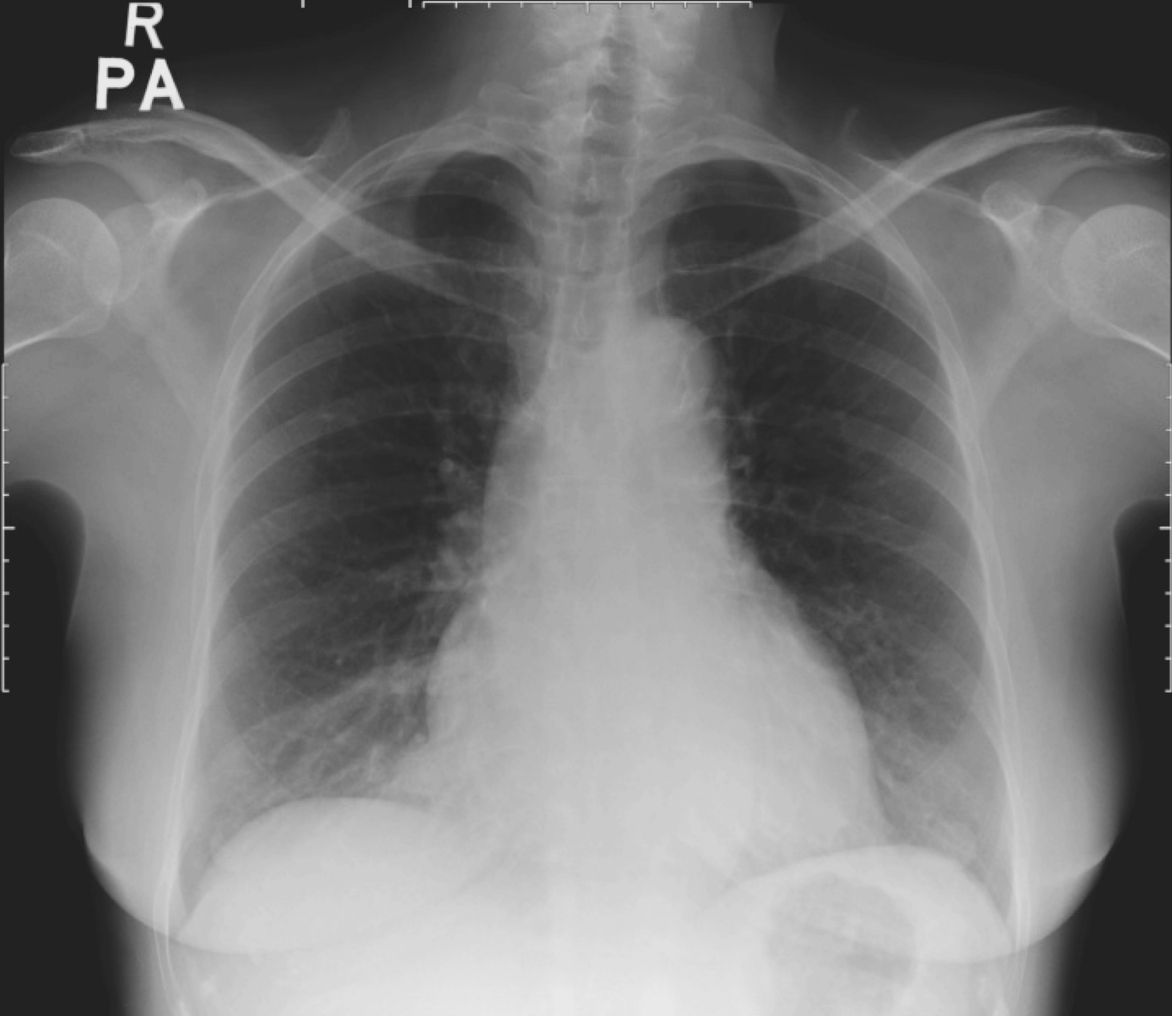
Repeated CXR four months after levothyroxine treatment revealing a significant reduction of cardiac diameter CXR: chest X-ray
